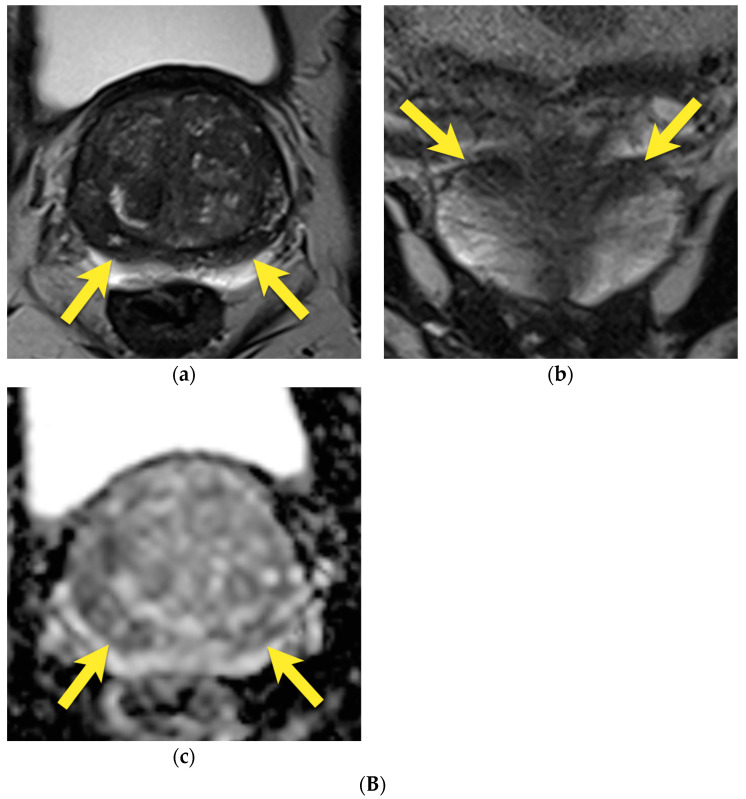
(A) Normal central zone: (a) axial T2-w image shows homogenously hypointense, symmetric area at the base of the prostate (yellow arrows), surrounding ejaculatory ducts (white arrows); (b) coronal T2-w image shows homogenously hypointense, symmetric area in conical shape extending from the base of the prostate (yellow arrows) to verumontanum (white arrowhead); (c) with mildly increased signal on DWI b 2000 image (yellow arrows); (d) and mildly decrease on ADC map (yellow arrows); (e) and diffuse little enhancement of progressive type (yellow arrows). (B) Patient with enlarged TZ due to BPH. The central zone in this patient is compressed between enlarged TZ and normal PZ and displaced towards the base of the prostate—the so-called “moustache sign”: (a) axial and (b) coronal T2-w images show homogenously hypointense, symmetric area at the base of the prostate (arrows); (c) with mildly decreased signal on ADC map (arrows).
